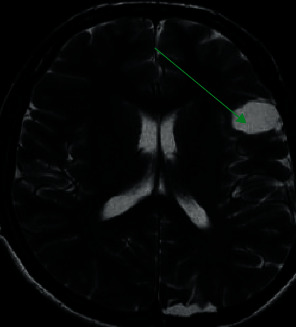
A brain MRI image of one male patient (62 years old) (the blue arrow marked the part of the lesion).
